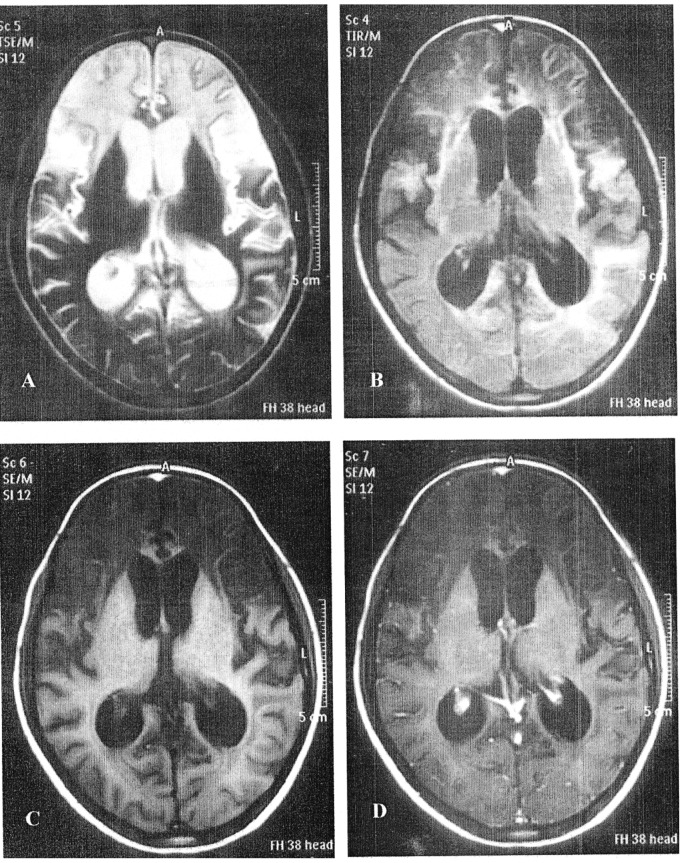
Magnetic resonance imaging of the brain of a 13-year-old boy previously treated with cranial irradiation. Diffuse bilateral confluent cortical and subcortical areas of abnormal attenuation are visible in both cerebral hemispheres, mainly in the frontal and parietal areas. High signal intensity was observed on (A) T2W1 and (B) FLAIR and low signal intensity was observed on T1W1 (C) without contrast and (D) with intravenous contrast. The images are consistent with grade III leukoencephalopathy. FLAIR, fluid attenuated inversion recovery; T2W, T2-weighted; T1W, T1-weighted.
